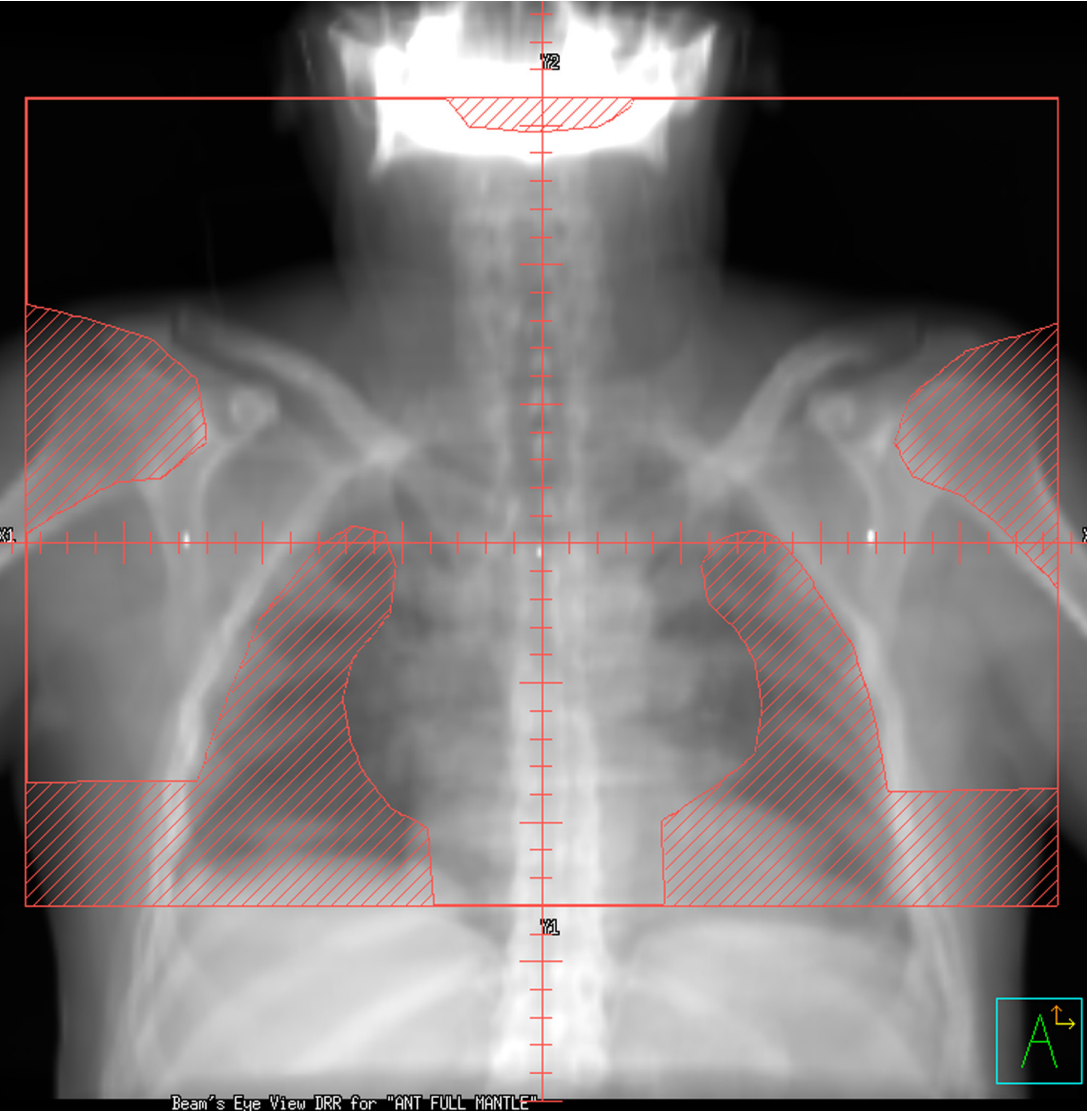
Digitally reconstructed radiographs demonstrating: mantle RT field (anterior beam shown).
